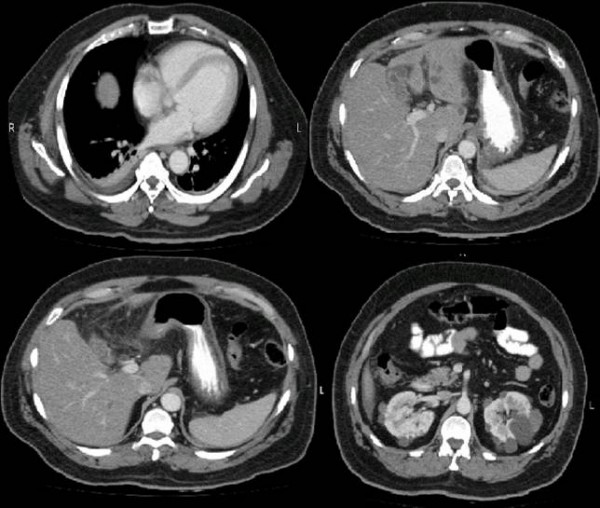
Cross sectional view of abdominal CT scan showing indistinct margins of gallbladder, pericholecystic fat stranding and streaks of fluid.
